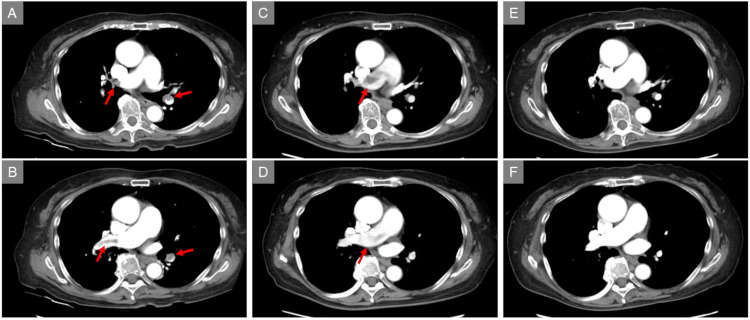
Serial CT images Contrast-enhanced CT revealed filling defects in both pulmonary arteries, consistent with thrombi (A and B, arrows). Follow-up CT revealed a filling defect consistent with newly formed thrombus in the main pulmonary artery (C and D, arrows). Outpatient follow-up CT confirmed complete resolution of the thrombus (E and F). CT, computed tomography
